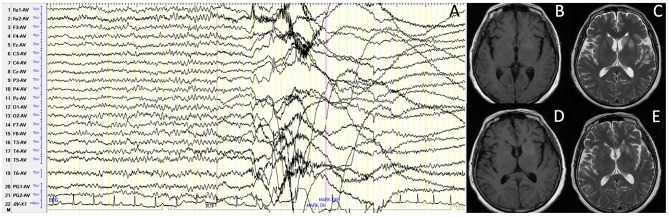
Case 9, female, 63 years old, who was diagnosed with leucine-rich glioma-inactivated 1 protein (LGI1) antibody-associated autoimmune encephalitis (AE). (A) Ictal electroencephalogram (EEG) of faciobranchial dystonia seizure (FBDS) showed that 1 s before the clinical onset, the amplitude of all the leads suppressed, followed by artifact of movements, which continued for 5 s, and then recovered to background. (B,C) Nineteen days after onset and before immunotherapy, brain MRI showed high T1/T2 signal on the left basal ganglia (caudal nucleus and lenticular nucleus). (D,E) Thirty-three days after onset (10 days after immunotherapy), brain MRI showed significant improvement in the high T1/T2 signal on the left basal ganglia.
